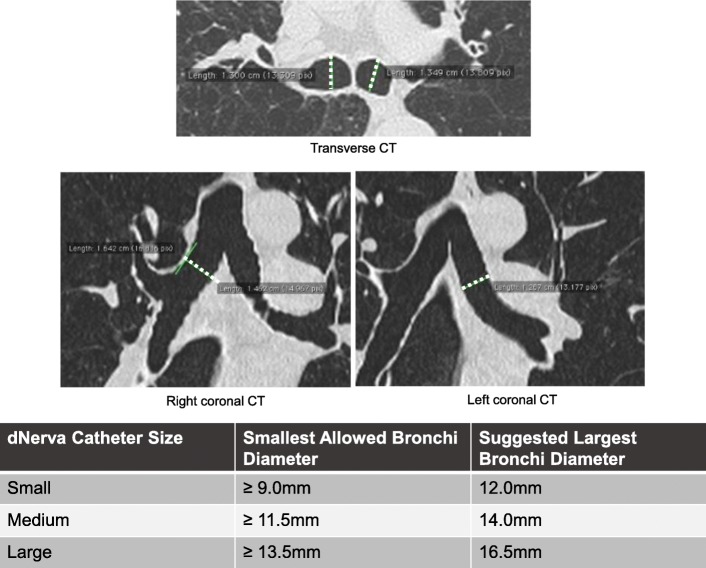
Example of transverse and coronal slice CT airway measurements. The patient’s computed tomography scan of the chest is reviewed prior to the procedure to re-confirm proper airway sizes and geometry. The right mainstem bronchial length must be ≥10 mm to ensure an adequate location for electrode placement. The diameters measured on the transverse scan and coronal scan (diameter indicated by white dotted lines on CT images) must be averaged to determine the appropriate catheter size for both mainstem bronchi
